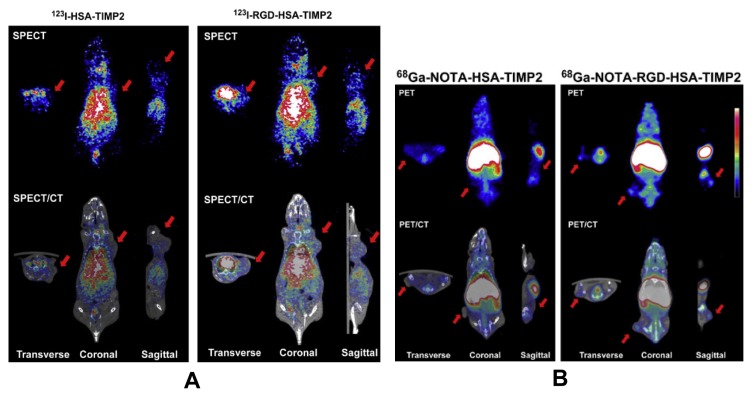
The SPECT and SPECT/CT images of ^123^I-HSA-TIMP2 (A, left) and ^123^I-RGD-HSA-TIMP2 (A, right) and the PET and PET/CT images of ^68^Ga-NOTA-HSA-TIMP2 (B, left) and ^68^Ga-NOTA-RGD-HSA-TIMP2 (B, right). Red arrows indicated the U87MG tumor. Reprinted with permission from [21].
